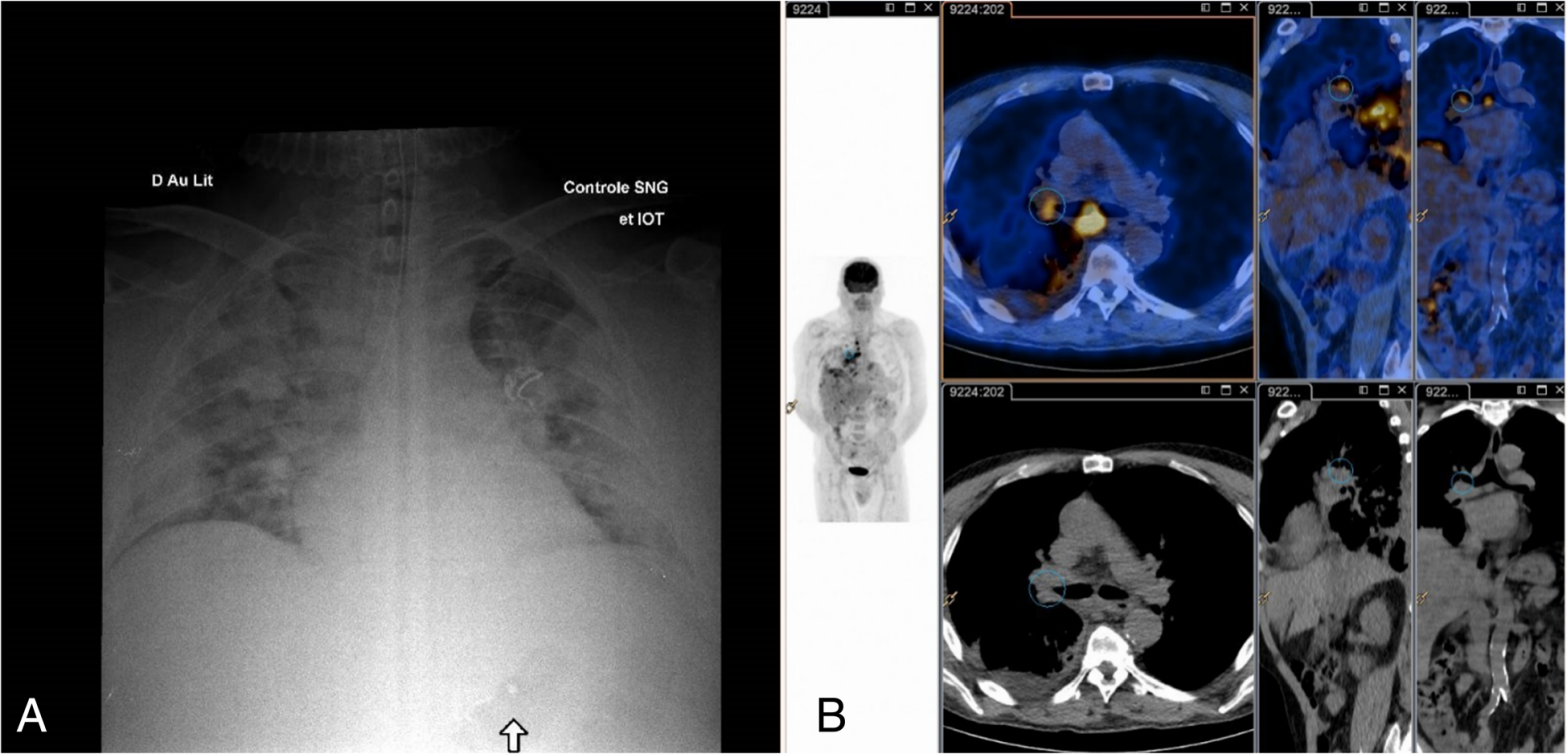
Thoracic imaging showing pulmonary lesions in systemic form of tularemia in a solid organ transplant recipient. a Thoracic X-ray showing bilateral alveolar and interstitial opacities during an acute respiratory distress syndrome (Case 2). b PET-scan (FDG-F18) showing pulmonary hyperfixations (left inferior lobe) and hypermetabolic mediastinal adenopathies (Case 1)
